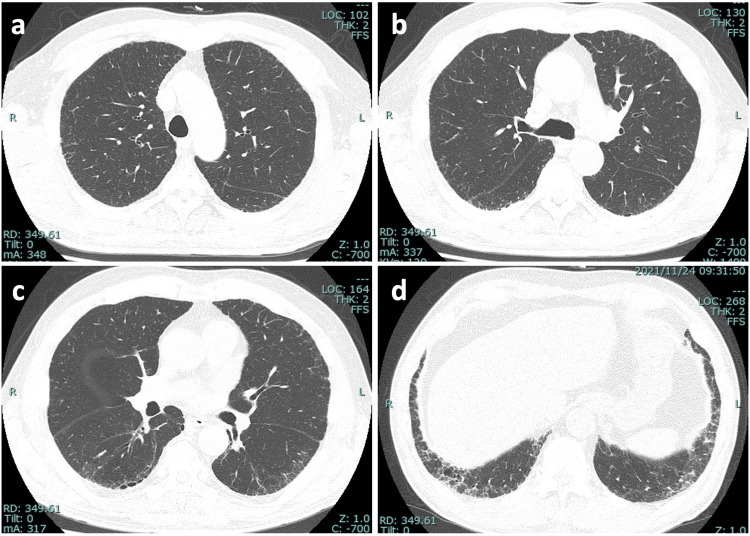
Chest CT images of the patient two years before admission. (a-d) A high-resolution chest CT scan taken two years prior to the patient's admission reveals a mild, subpleural, and basally predominant reticular pattern, accompanied by traction bronchiectasis and bronchiolectasis across both lungs, indicative of a probable UIP pattern. UIP: usual interstitial pneumonia
